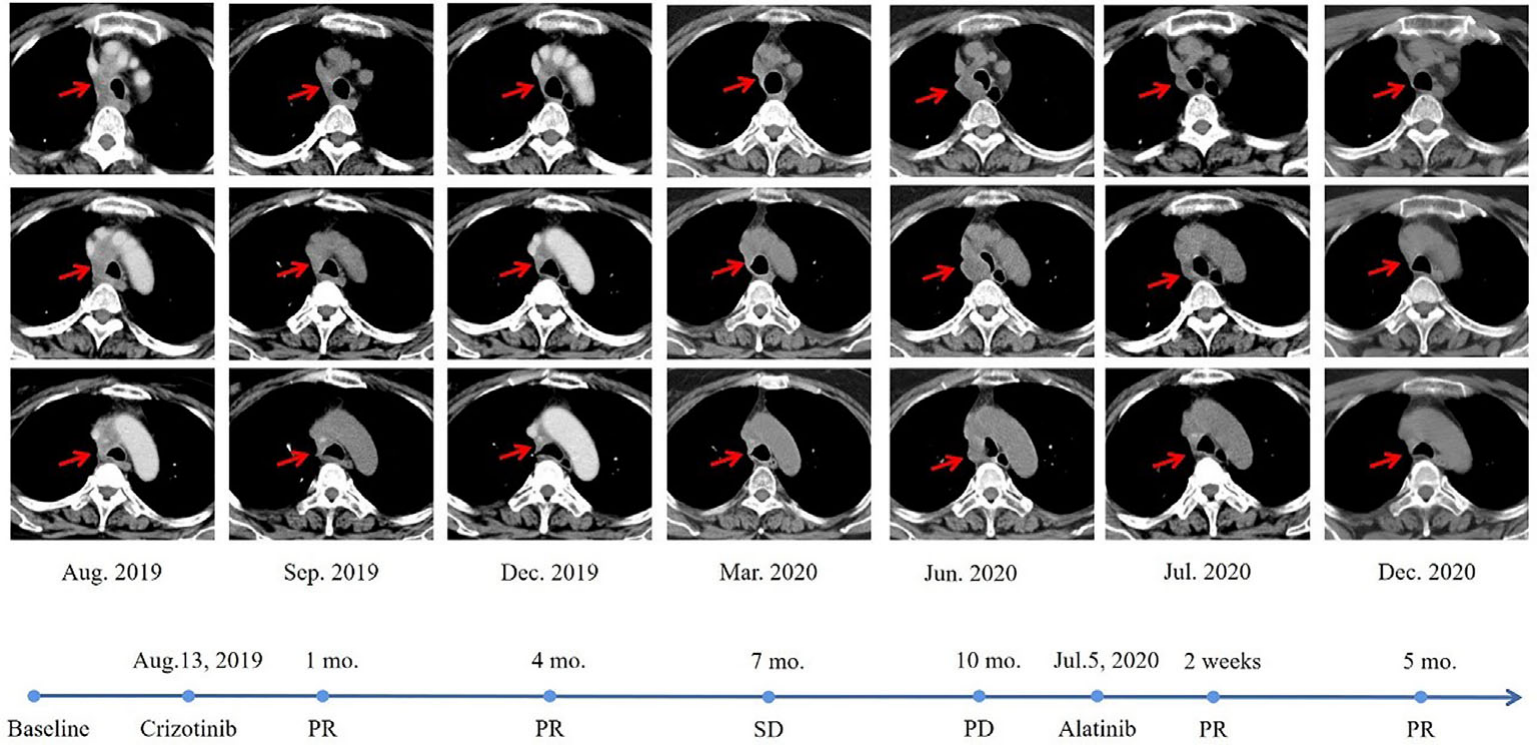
Radiological evaluation before and after therapy. The CT scan images demonstrated a significant reduction in pulmonary lesions.
